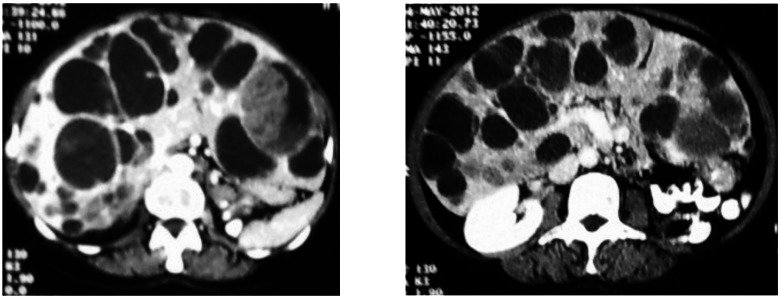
Contrast computed tomography (CT) scan reveals massive hepatomegaly and a polycystic liver with multiple cystic formations.
